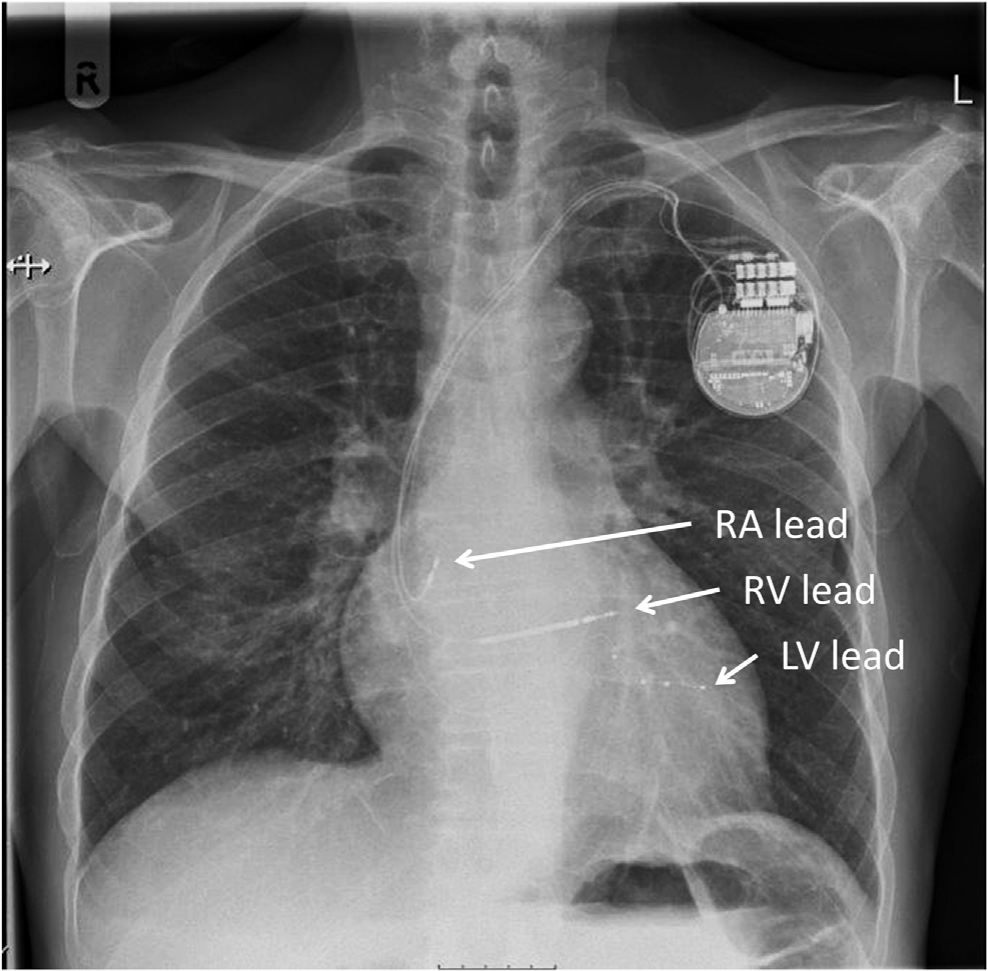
Chest radiograph displaying device and lead positions. This posteroanterior projection shows the final positions of the device and leads. Left ventricular (LV) lead was placed at the basal-mid posterolateral branch of the coronary sinus, right ventricular (RV) lead at the RV apex, and right atrial (RA) lead in the RA appendage.
